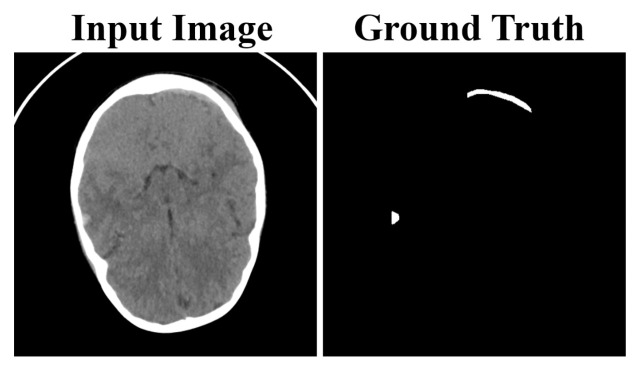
Examples of ICH CT images with corresponding semantic segmentation annotations.
